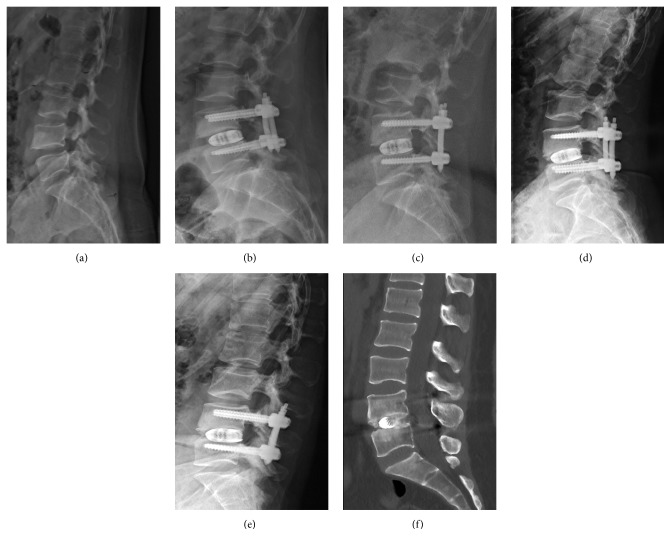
(a) Preoperative lateral radiograph showing isthmic spondylolisthesis. (b) and (c) Lateral radiograph at 1 and 12 months postoperative. (d) and (e) Extension and flexion lateral X-rays at two-years follow-up. (f) Sagittal CT image obtained 2 years postoperatively showing interbody fusion.
